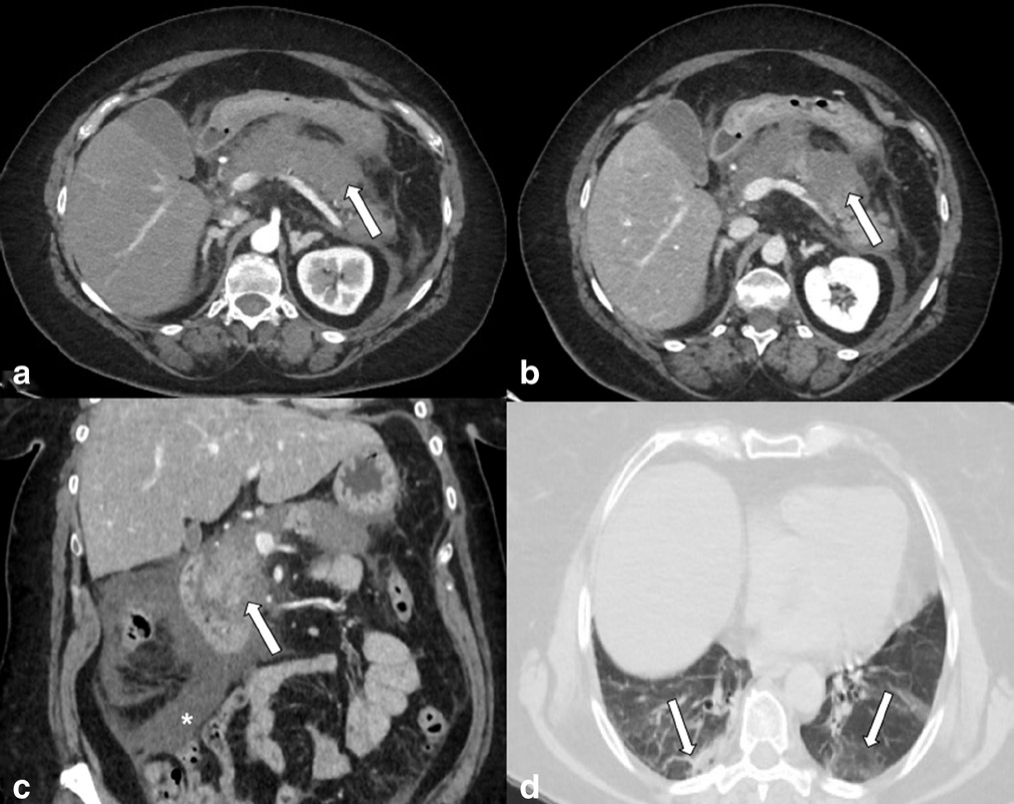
Enhanced CT scan. (a) Arterial phase, Axial image, (b) Portal phase, Axial
